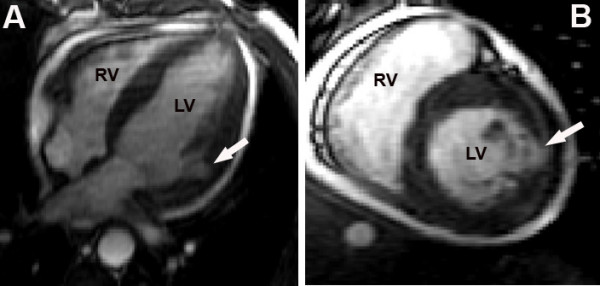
Magnetic resonance imaging in the apical four-chamber view (A) and short axis view (B). The arrows points to the presence of contrast in myocardium similar to that seen by contrast echocardiography. LV = left ventricle; RV = right ventricle.
